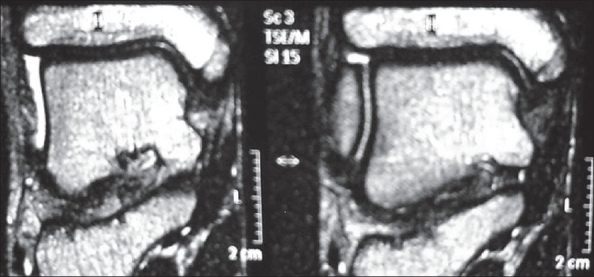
T2 WI MRI scan (coronal image) of the right ankle shows homogenous intensity of the accessory center
